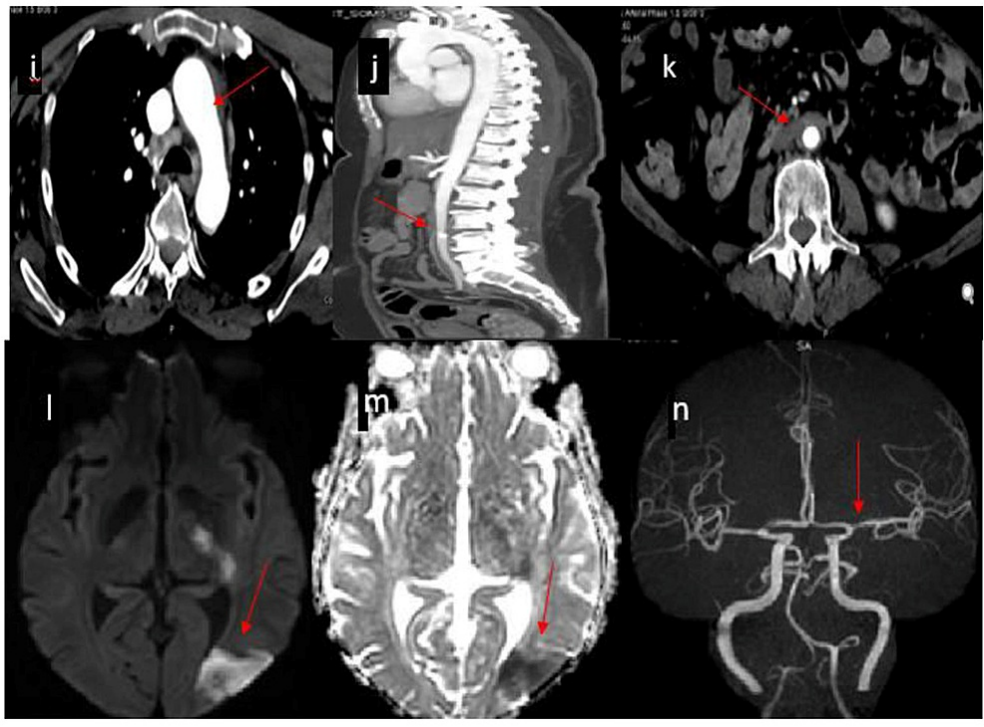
Repeat CT aortic angiography showed diffuse atherosclerotic changes in the aortic arch, descending thoracic and abdominal aorta. These atherosclerotic changes are most severe at the distal part of the descending thoracic aorta which showed circumferential mural thickening and calcification (j, k). No evidence of intraluminal filling defects or floating thrombi (i-n). MRI/MRA head showed left posterior parieto-occipital, left centrum semiovale, left thalamocapsular and left peritrigonal region acute ischemic infarction and left side MCA-M1 segment showed moderate stenosis with mild stenosis proximal basilar artery (l, n). MCA: Middle cerebral artery
